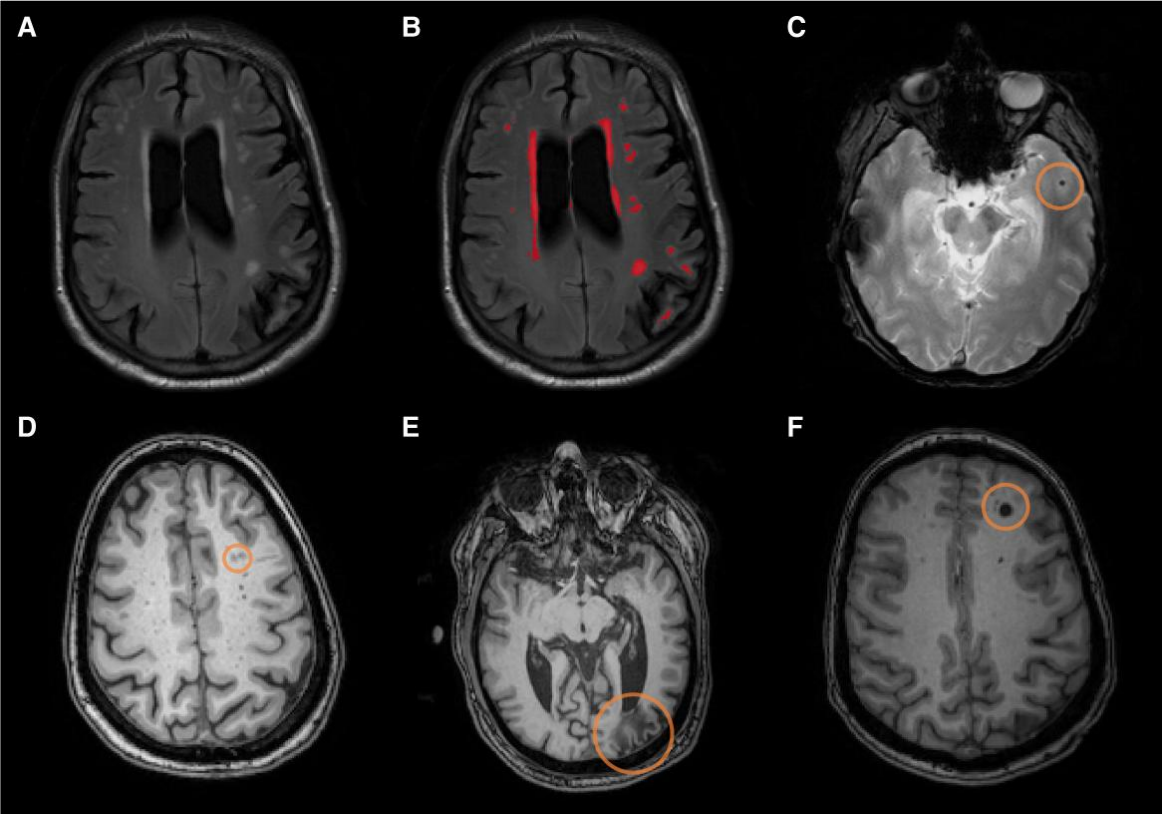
MRI markers of cerebrovascular disease included in the study. (A) Unlabelled WMH on an axial FLAIR image. (B) Labelled WMH in red on an axial FLAIR image. (C) Microbleed circled on an axial T_2_*-GRE. (D) Perivascular spaces circled on an axial T_1_-weighed image. (E) Distal cortical infarct circled on an axial T_1_-weighted image. (F) Deep infarct circled on an axial T_1_-weighted image.
